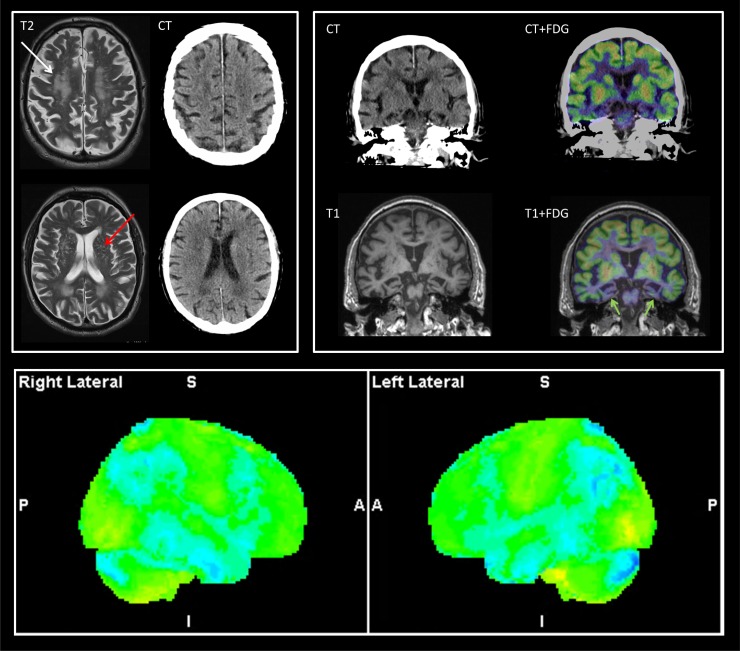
Minor clinical impact. CT and MRI performed 10 days apart. T2 MRI shows more pronounced white matter lesions (white arrow) compared to CT (Fazekas score 3 vs 2) and demonstrates also widening of perivascular spaces in the basal ganglia (red arrow) often considered a sign of vascular disease (état criblé). T1 MRI showed more pronounced atrophy of mesial temporal lobe compared to CT (upper right panel) scored as MTA 3 vs 2 on CT. FDG PET shows parietotemporal hypometabolism with involvement also of mesial temporal structures (green arrows, upper right panel) suggestive of Alzheimer’s disease. With MRI, classification of PET changed from neurodegenerative disease to neurodegenerative + vascular disease and the clinical diagnosis changed from neurodegenerative disease to mixed dementia. As the change did not prompt change of therapy, but only diagnosis, the change was classified as minor impact.
